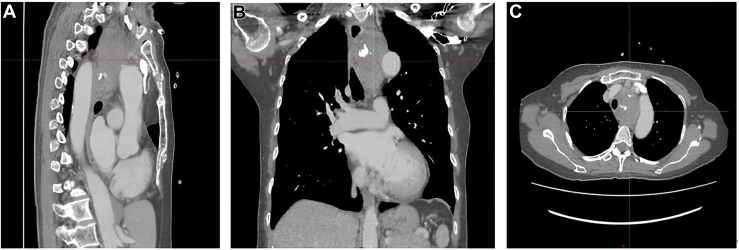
Contrast-enhanced computed tomography showing a residual cervicomediastinal thyroid mass (intrathoracic goiter) with mediastinal extension and mass effect on adjacent structures. (A) Sagittal view demonstrating the craniocaudal extent of the mass. (B) Coronal view highlighting the intrathoracic goiter. (C) Axial view demonstrating the lesion and its relationship to the trachea and surrounding mediastinal structures.
